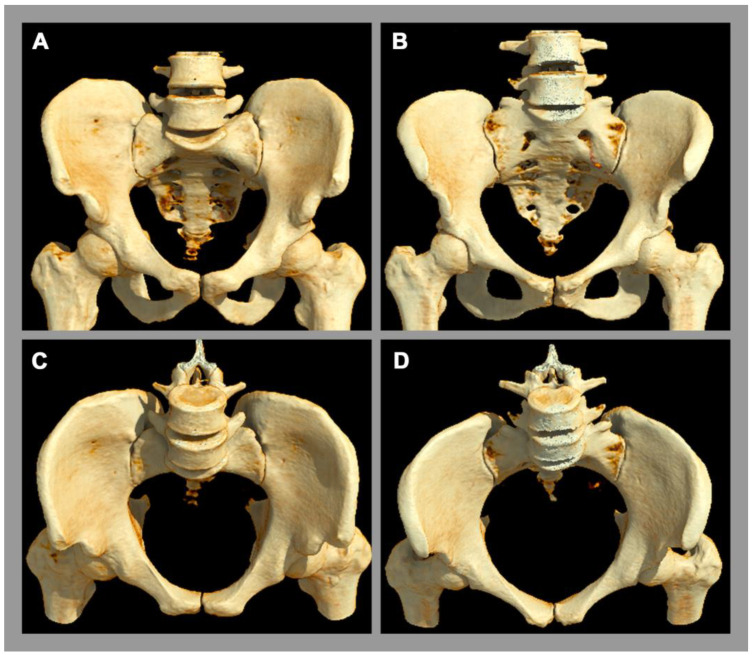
Anatomy of the pelvis. A 3D computed tomography reconstruction. (A,C) Male pelvis. (B,D) Female pelvis. There is obvious sexual diamorphism in the pelvic anatomy. The male pelvic cavity is longer and more conically configurated with a longer and narrower sacrum, while the female pelvic cavity is narrower and cylindrically orientated.
